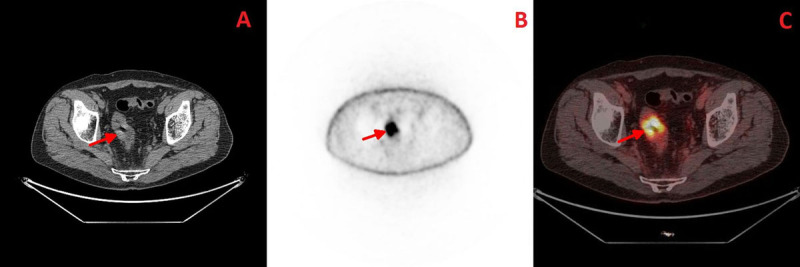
Axial plane; A- CT image highlighting the rectal tumour; B- PET image; C- Fused PET-CT image.
